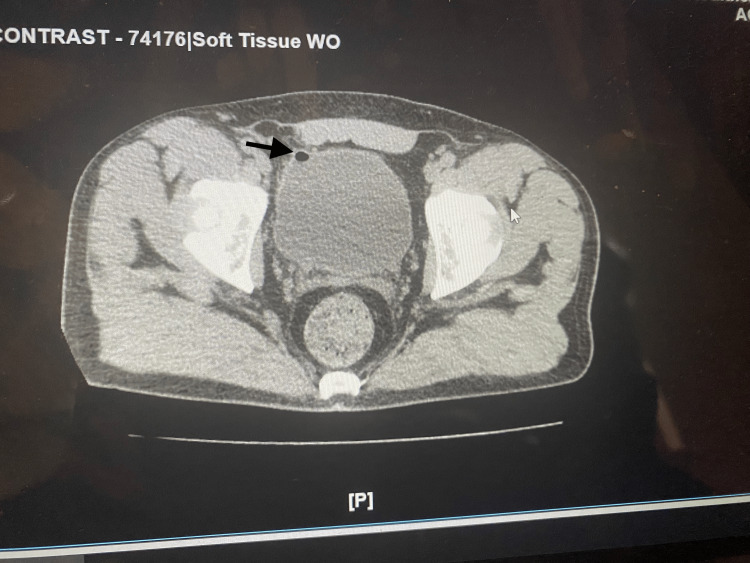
CT of abdomen/pelvis with intravenous contrast. The black arrow shows the colovesical fistula with air in the bladder.
